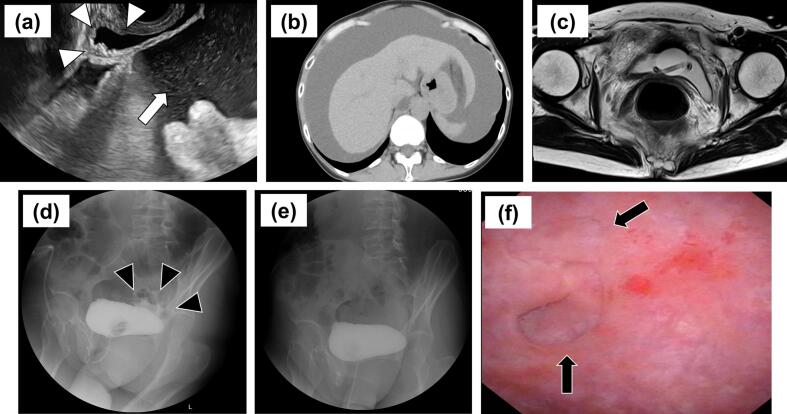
Imaging findings of bladder rupture at the age of 55 years. (a) Transvaginal ultrasonography shows a moderate amount of urine retention in the bladder (white triangles) and a large amount of peritoneal fluid (white arrow). (b) Computed tomography shows peritoneal fluid from below the diaphragm to the pelvic cavity. (c) MRI T2-weighted image shows no lesion of endometriosis in the bladder. (d) Retrograde cystography shows minor contrast leakage from the posterior wall of the apex of the bladder (black triangles). (e) Cystogram on day 9 after admission shows no contrast leakage. (f) On day 10, cystoscopy shows bladder diverticulum caused by previous cystectomy (black arrows).
